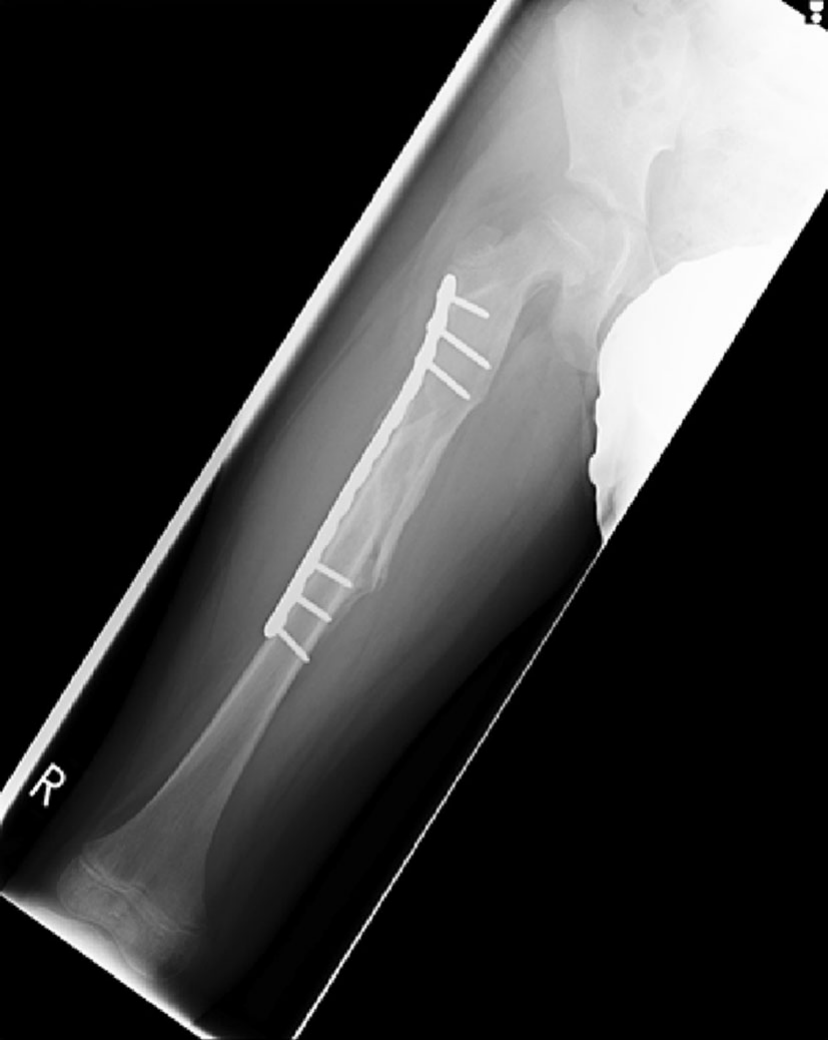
AP radiograph showing the patient from Fig. 8, treated with a submuscular 3.5-mm bridge plate. At just 8 weeks, there is abundant callus and the patient is fully weight bearing
